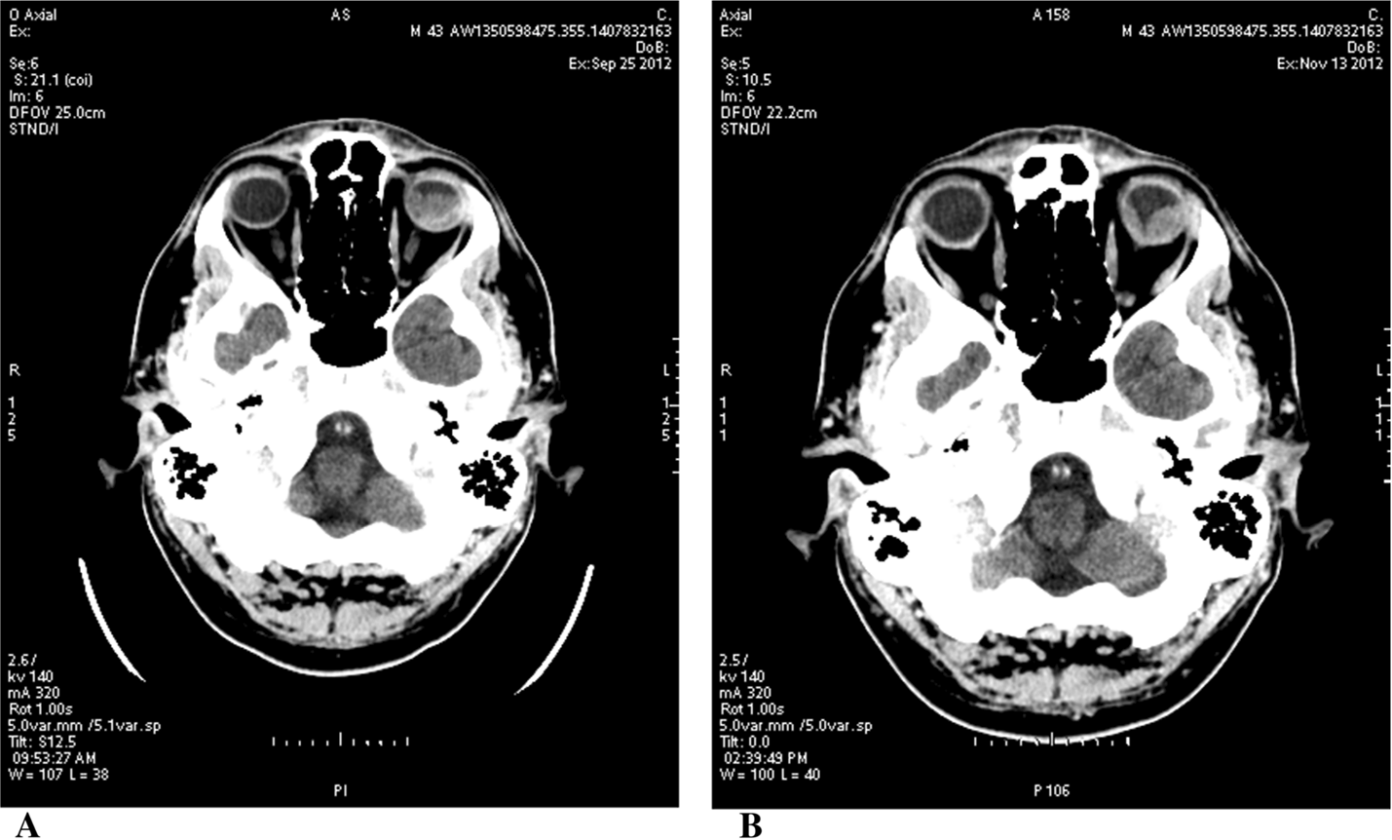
Basal CT-scan of the eyes (A) and after 45 days on crizotinib (B), showing a reduction of the abnormal tissue in the left eye.
